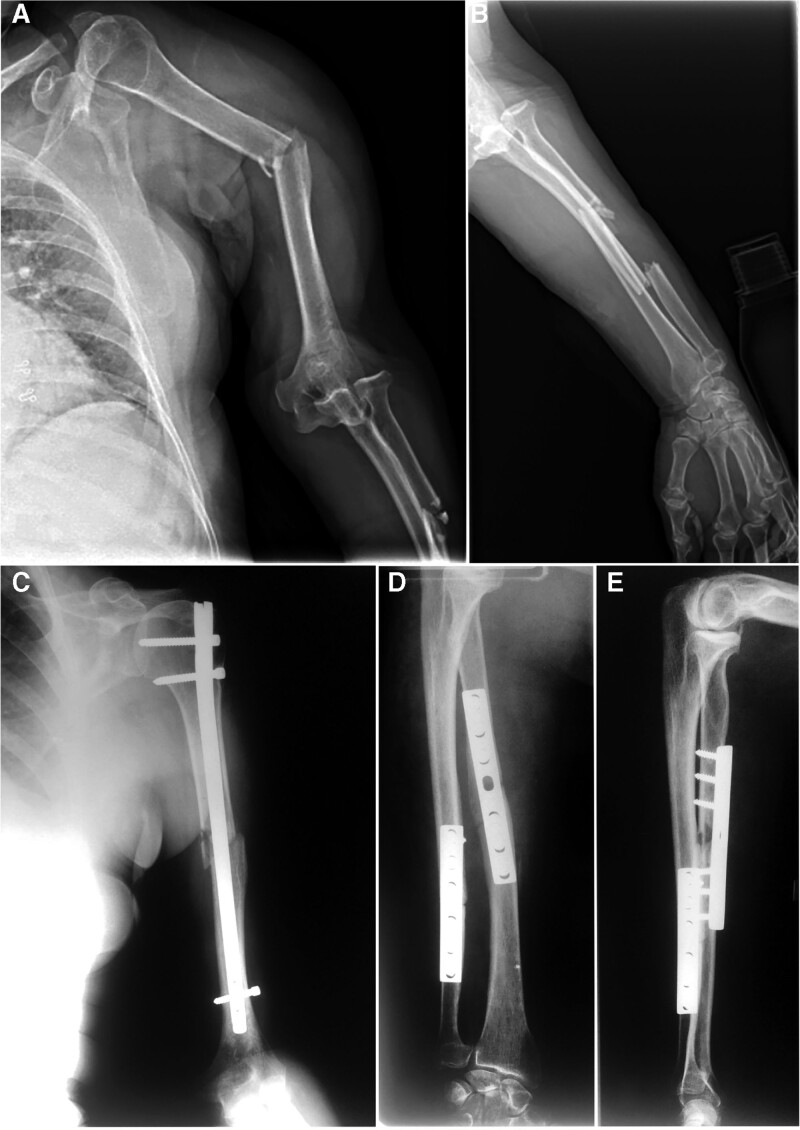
(A) Initial X-ray (antero-posterior view) of the arm – displaced middle third humeral shaft fracture and postero-lateral elbow dislocation. (B) Initial X-ray (lateral view) of the forearm – proximal third radial shaft and distal third ulnar fractures, and posterior elbow dislocation. (C) Postoperative X-rays (antero-posterior view) of the arm – humeral shaft fracture reduction and fixation with a locked intramedullary nail. (D, E) Postoperative X-rays (antero-posterior and lateral views) of the forearm – reduction of fractures and fixation with plates, reduction of the elbow dislocation.
